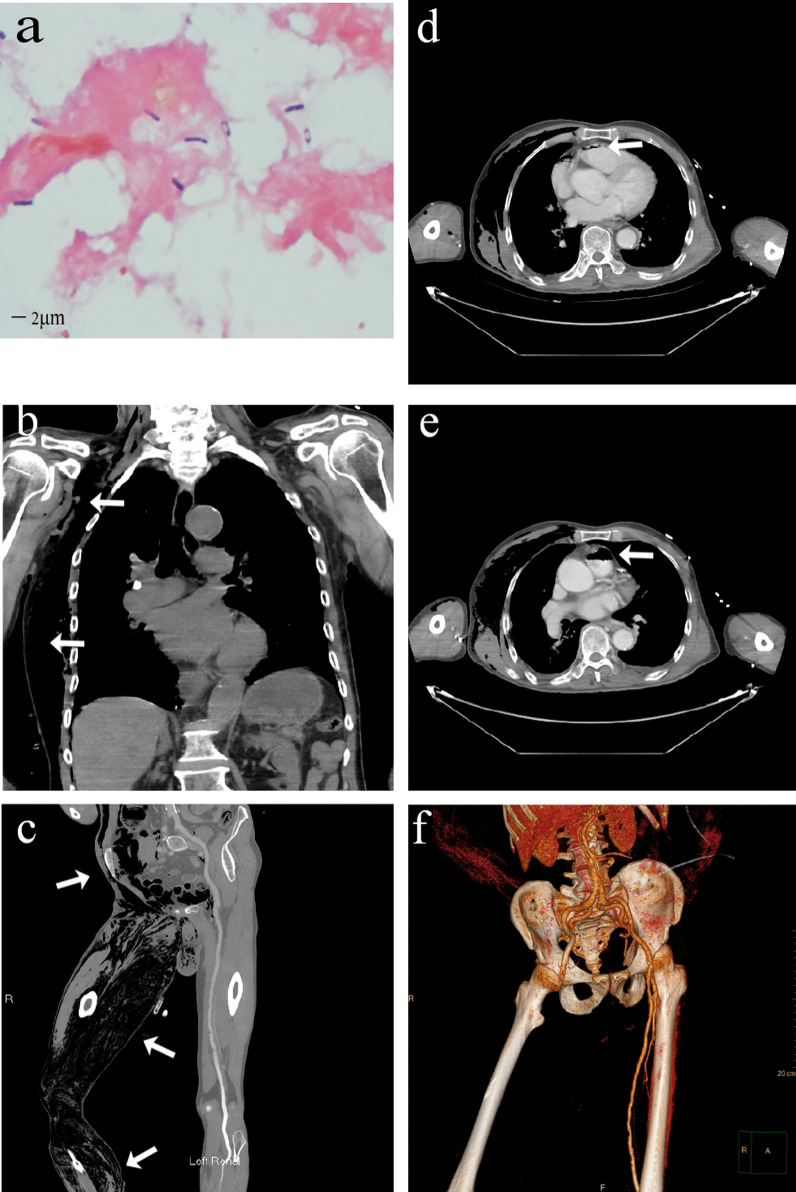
Bacterial smear and CT images. a Gram-positive bacilli on bacterial smear. b Pneumatosis in the right side of the neck, chest wall, upper abdomen (shown by the white arrow). c Pneumatosis in the right lower abdomen and lower extremities (shown by the white arrow). d Pneumatosis in the right ventricle (shown by the white arrow). e Pneumatosis in the pulmonary artery (shown by the white arrow). f CTA reconstruction shows occlusion of the right femoral artery. *CT* computerized tomography, *CTA* computerized tomography angiography
